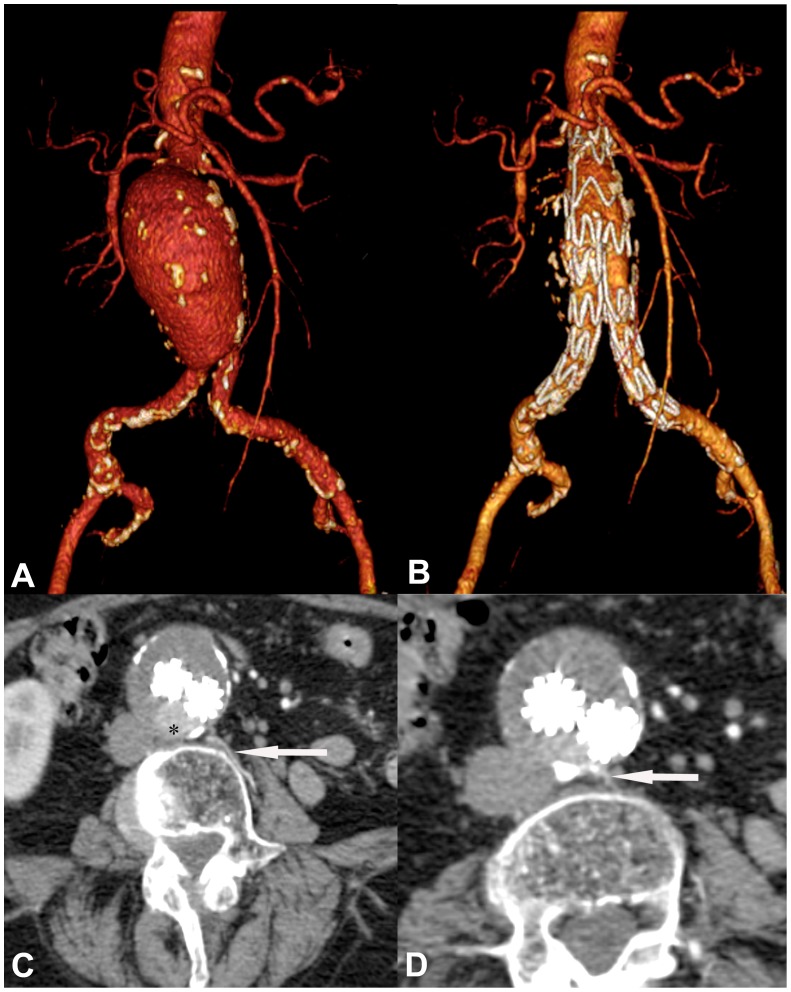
CTA of a large infrarenal AAA in a 79-year-old patient. A, Volume reconstruction (VR) of CTA with moderate calcification within the aneurysm sac and both common iliac arteries. B, VR performed 12 days after implantation of a Talent stent graft. C, Venous phase CTA reveals a type 2 endoleak (asterisk) posterior of the stent graft limb and a perfused lumbar artery on the left (arrow). D, More inferiorly, the site of entry of the lumbar artery into the aneurysm sac is seen (arrow).
